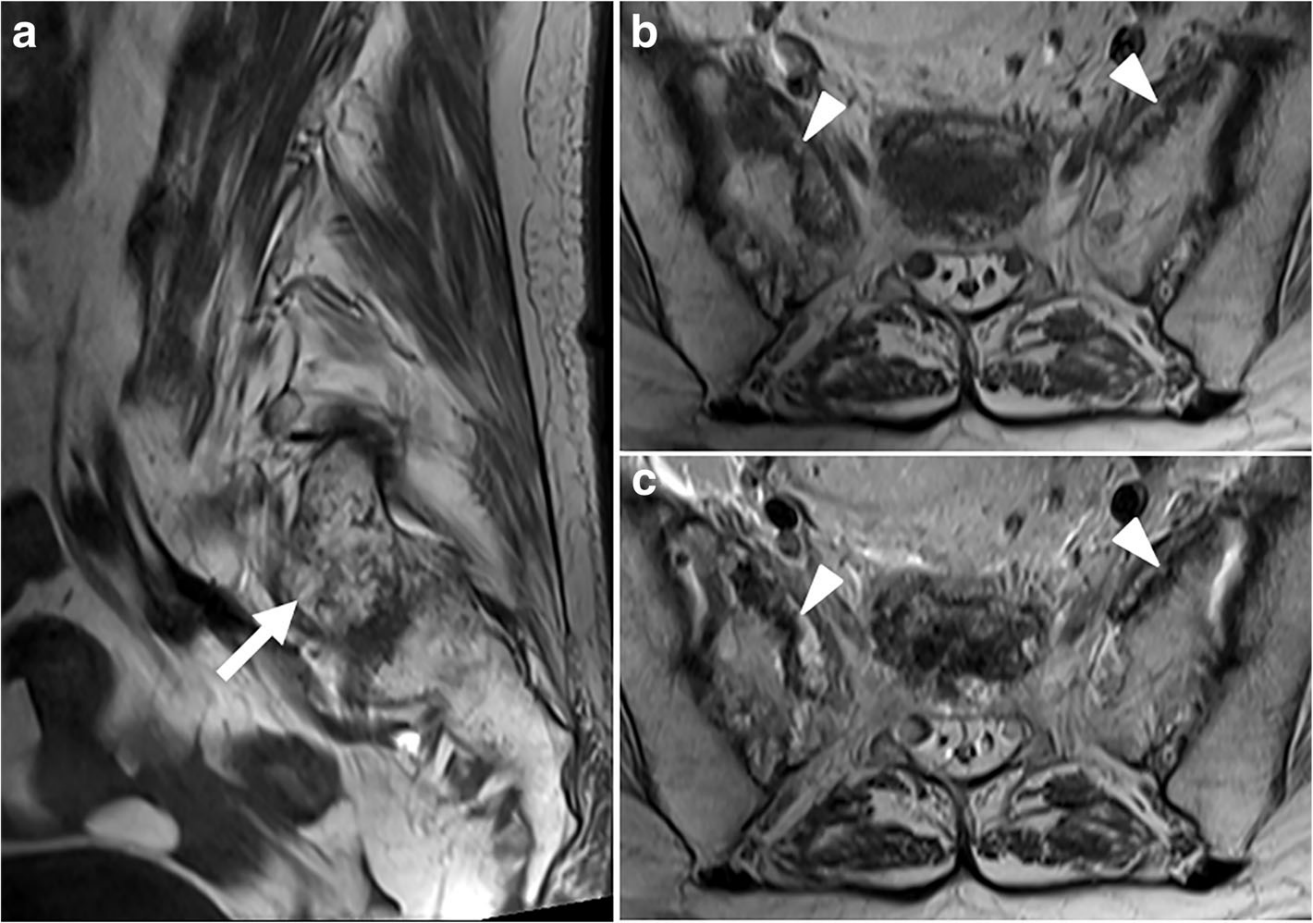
An 81-year-old woman with SIF involving the bilateral sacral alae with a horizontal component (not shown). a Sagittal T1-weighted MR image shows hypointense streaks at the right sacral ala (arrow). b and c, Axial T1-weighted (b) and T2-weighted (c) MR images at the distal S1 level show hypointense fracture lines at both sacral alae with minimal surrounding bone marrow edema (arrowheads)
